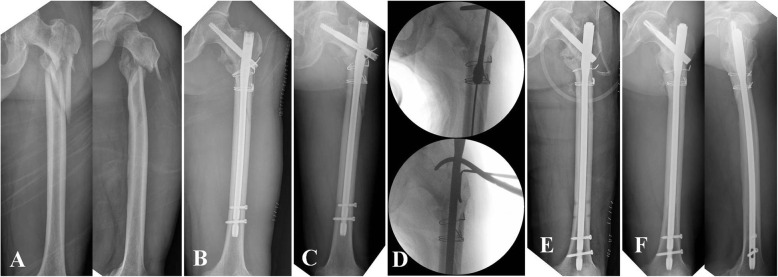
a A 52- year-old man who sustained reverse oblique fracture with subtrochanteric extension by high-energy injury. b Postoperative radiograph after open surgery. c Nonunion and implant failure at 14 months after initial surgery. d Revision surgery using closed procedure without bone graft. e Postoperative radiograph. f Bony union at 7 months after revision surgery
